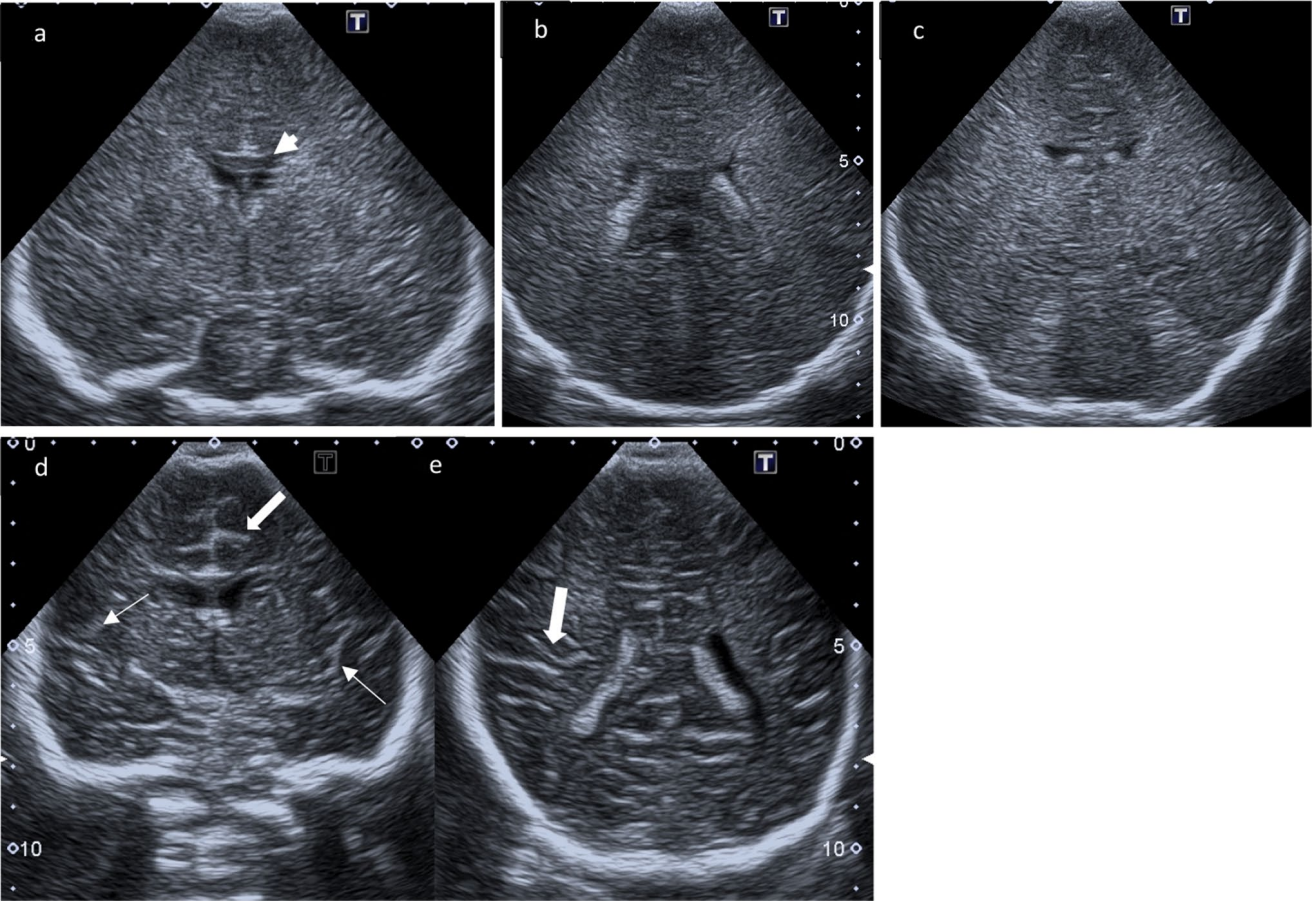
Coronal ultrasound scans. a–c Coronal scans through the foramen of Monro, the choroid plexus, and the posterior aspect of 3rd ventricle above the tentorium: generalized increased echogenicity of brain parenchyma associated with obliteration of the gyral-sulcal interfaces and narrowing of interhemispheric fissure; the Corpus callosum (arrowhead) remains hypoechoic. d, e Comparison to normal age-matched US: Coronal scans through the foramen of Monro and through the choroid plexus respectively with normal demarcation of Sylvian fissure (small arrows) and gyral-sulcal interfaces (large arrows)
